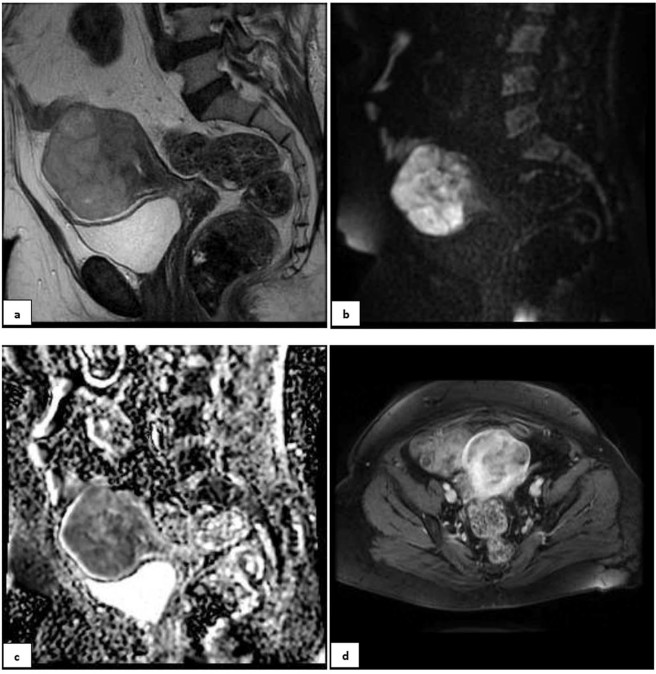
A 55-year-old postmenopausal patient with 3 months of abnormal uterine bleeding and a hypervascular myometrial mass on her ultrasound. (a) Sagital T2 MR-image detected a large predominantly hyper-signal lesion in anterofundal myometrium with extension to endometrial canal; Tumor-Myometrial Contrast (TM) Ratio = 1.98; T2-scaled Ratio = 1.01 and T2 map = 81. (b) Sagital-DW image and (c) ADC revealed restriction with mean ADC of 0.72 mm/s^2^. (d) Axial post contrast T1 image in equilibrium phase showed the mass has central necrosis and Tumor-Myometrium Contrast Enhanced (TMCE) Ratio = 1.1. If we put this sample data in either of simple or complex decision-tree, the pathology could be predicted as “malignant”. Eventually, the histo-pathological exam confirmed malignancy, a high grade sarcoma.
